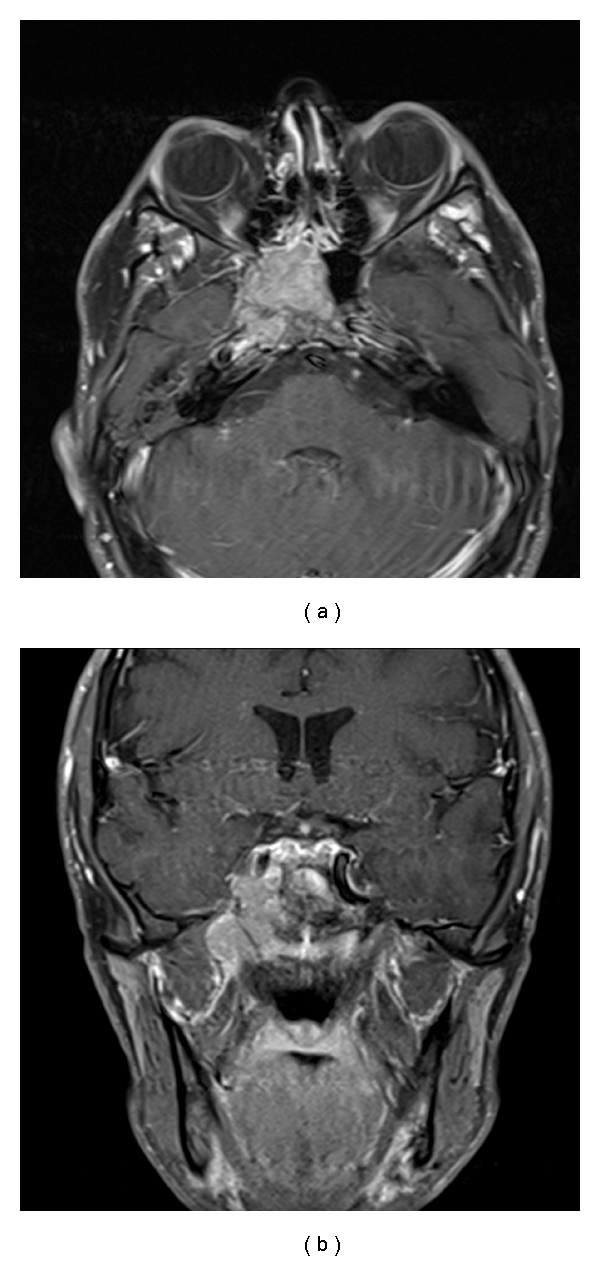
Axial and coronal T1-weighted, gadolinium-enhanced MR imaging revealing a homogenously enhancing lobulated lesion centered within the right sphenoid sinus with extension into the carotid canal, pterygopalatine fossa, and optic nerve canal.
